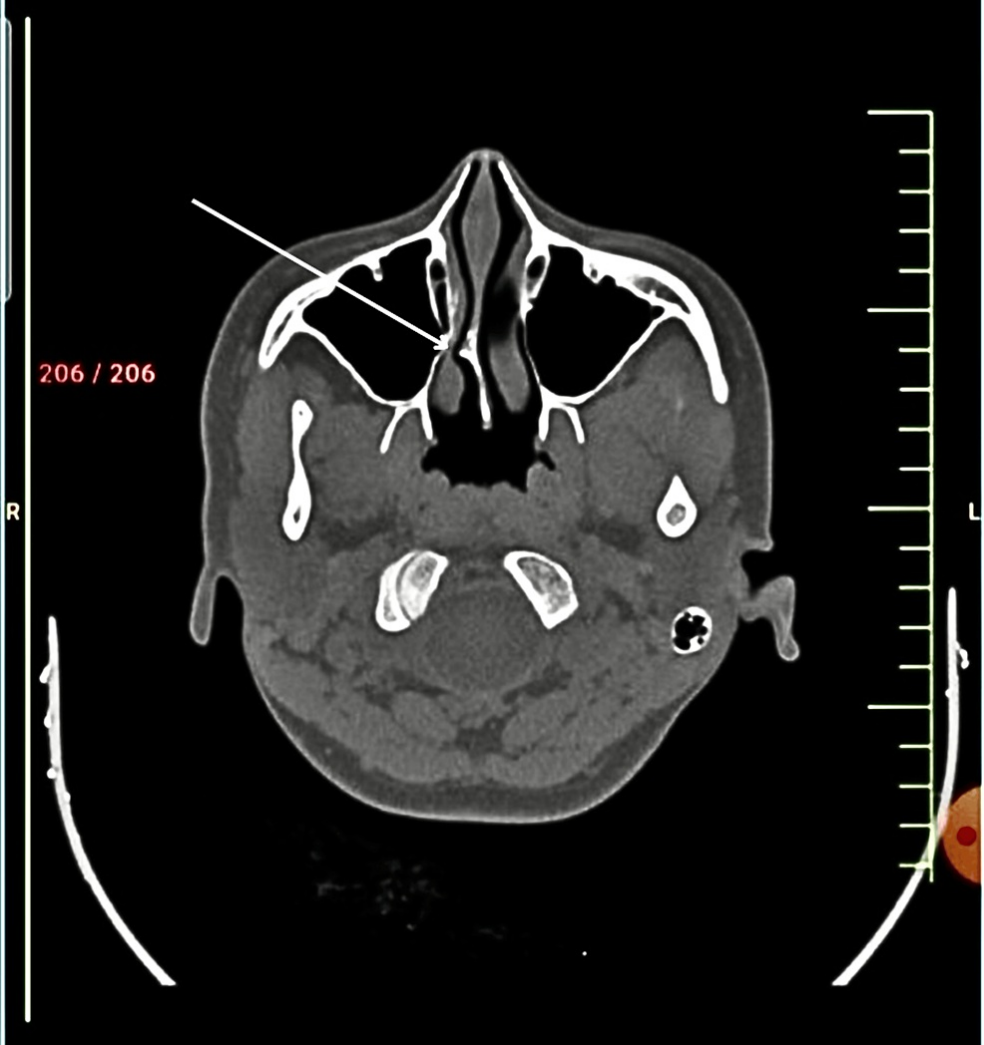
CT scan of the paranasal sinuses of the patient showing anterior nasal septum spur on the right side
